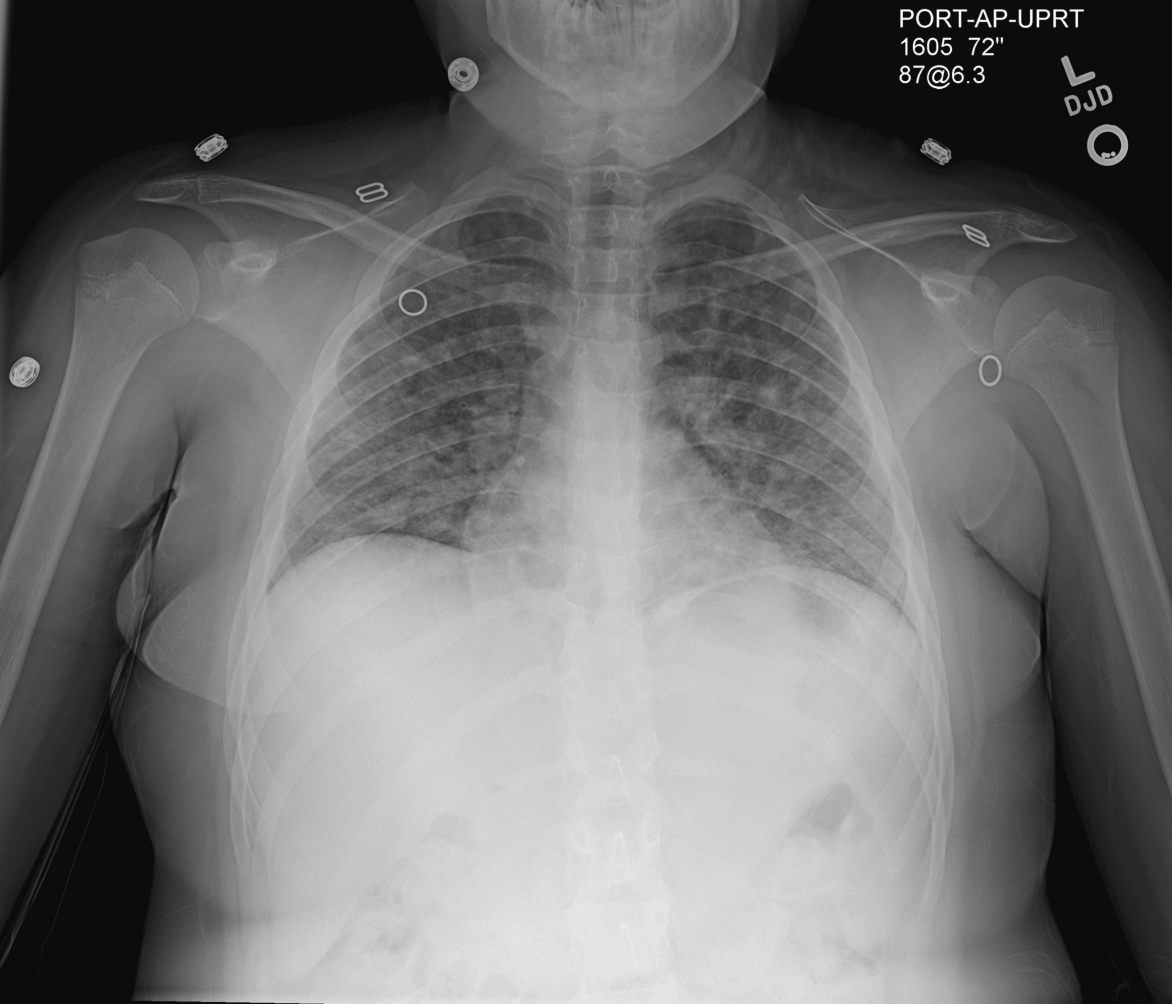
Emergency department chest X-ray
